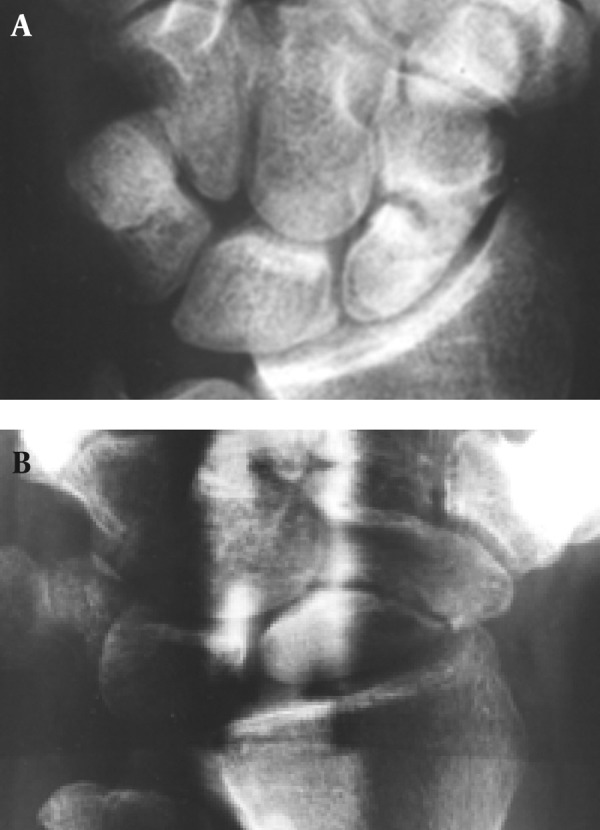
A 19-year-old man with wrist trauma. Conventional and panoramic radiography was performed 4 months after injury. A, Conventional radiograph shows doubtful union. B, Panoramic radiograph shows delayed union.
